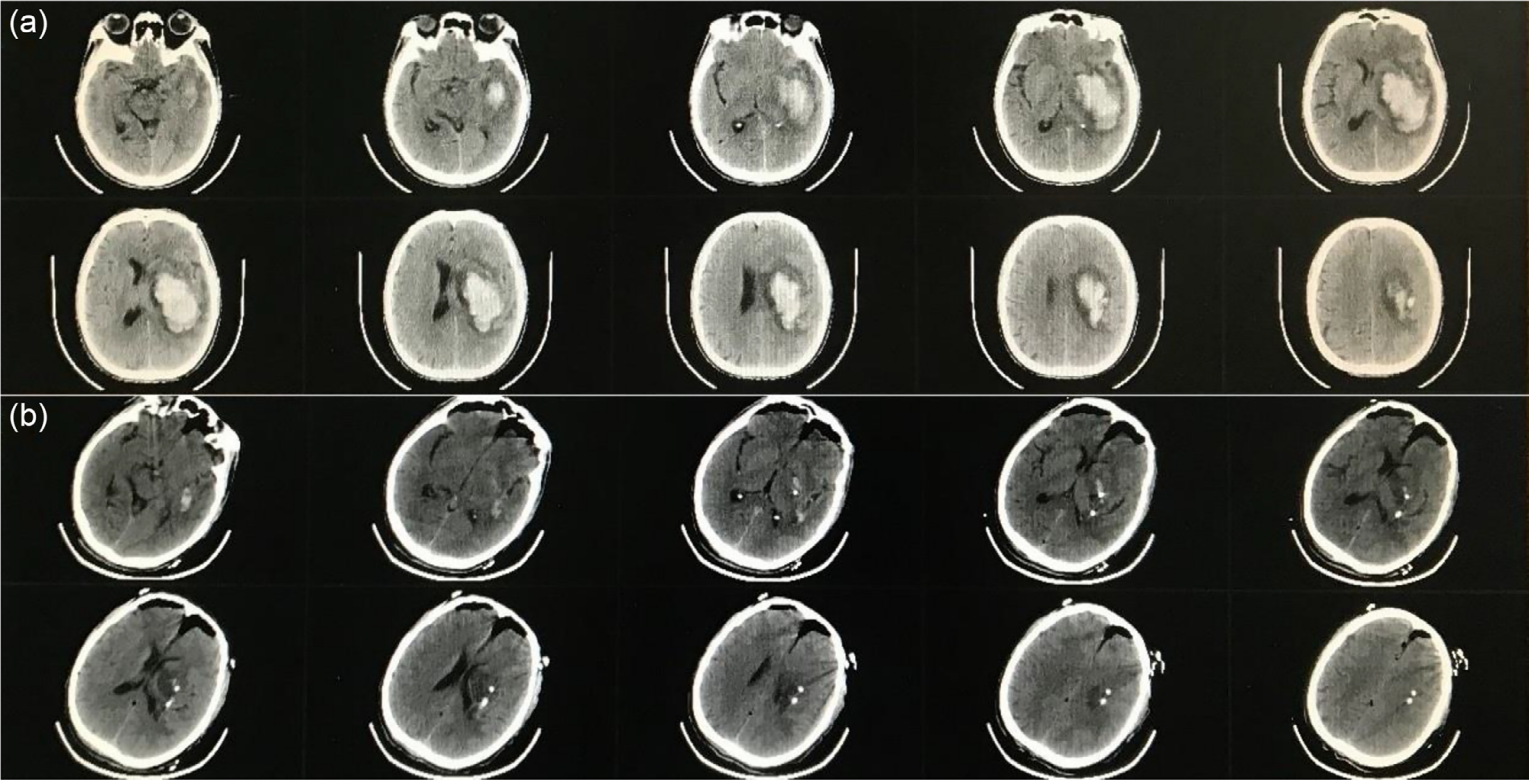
Head computed tomography (A) before and (B) after robot‐assisted minimally invasive stereotactic puncture therapy.
